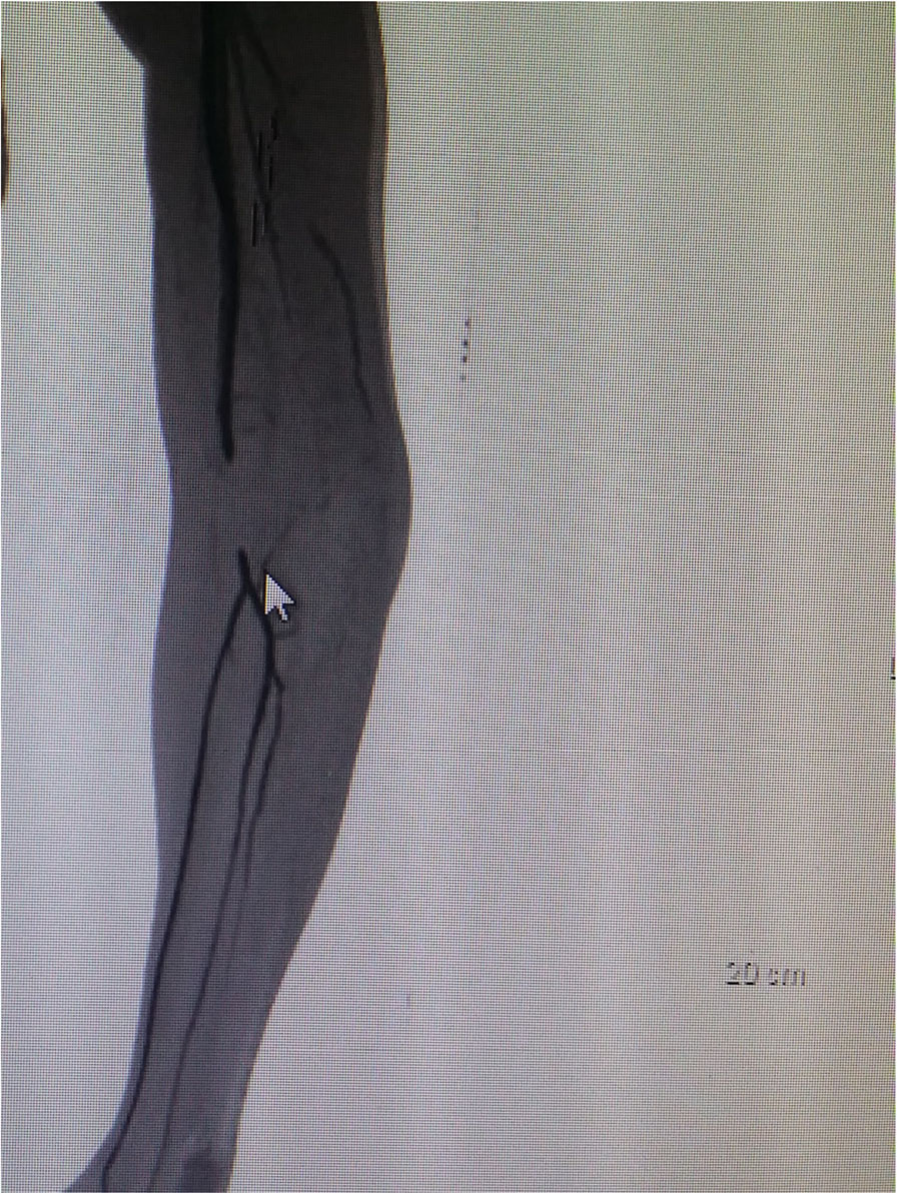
Computed tomography angiogram of left upper limb. Arrow indicating non opacification of brachial artery on CTA
